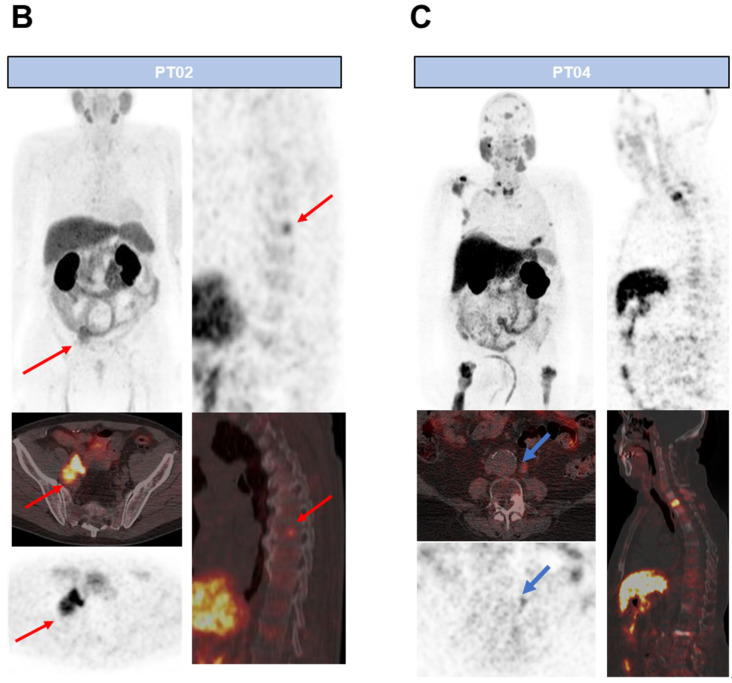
Study design and PET images. (A) Details of the PET study and summary of patients and lesions analysed. (B) Typical [^18^F]D4-FCH uptake. MIP, axial, and sagittal views of PET and PET/CT of PT02, showing right external iliac node and T8 bone metastasis (red arrows). (C) Typical [^18^F]D4-FCH uptake. MIP, axial, and sagittal views of PET and PET/CT of PT04, showing multiple bone metastases and a left paraaortic nodal metastasis (blue arrows).
